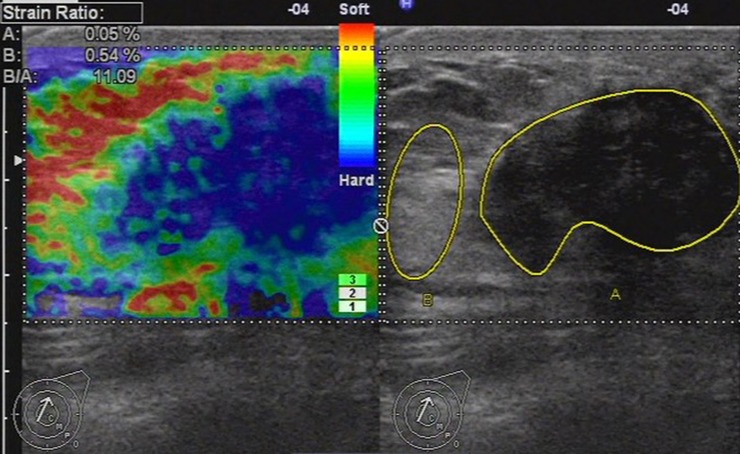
Ultrasonic elastographic image of an IDC in a 52-year-old woman. The lesion was scored 5 with the 5-point scoring method and the maximum diameter of the lesion was 28.1mm. The strain ratio was 11.09 with the strain ratio method at the same breast tissue depth as the reference.
